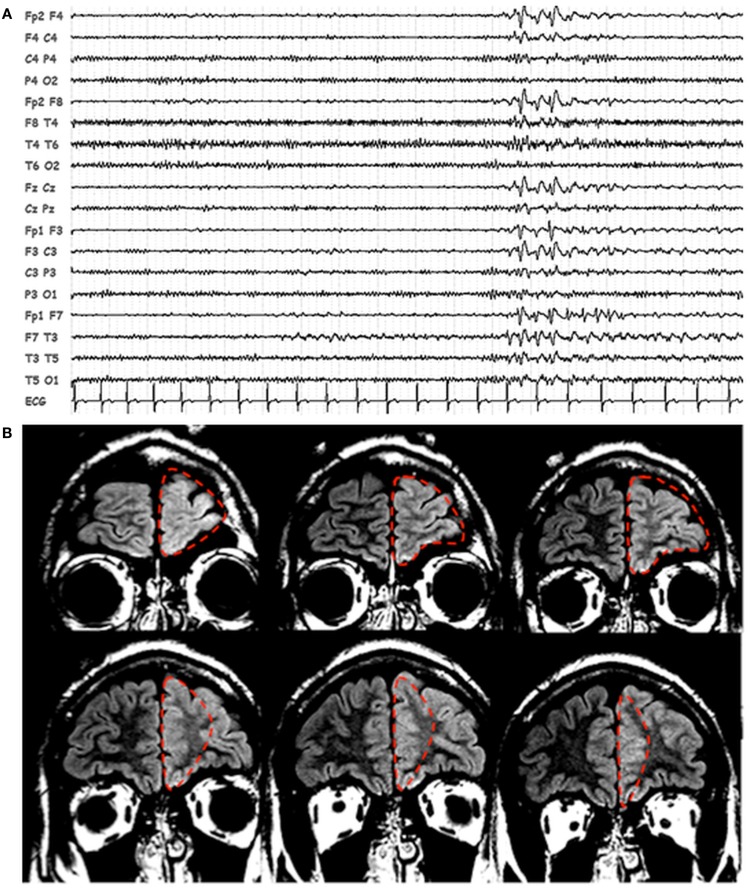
Patients’ EEG and structural MRI scan. (A) Representative page of scalp EEG interictal epileptiform abnormalities with bi-frontal/left frontal predominance. EEG is displayed in bipolar montage. (B) Fluid attenuated inversion recovery (FLAIR), coronal images showing thickening and blurring of the left prefrontal cortex. The dashed red line identified the boundaries of the first surgery resection.
